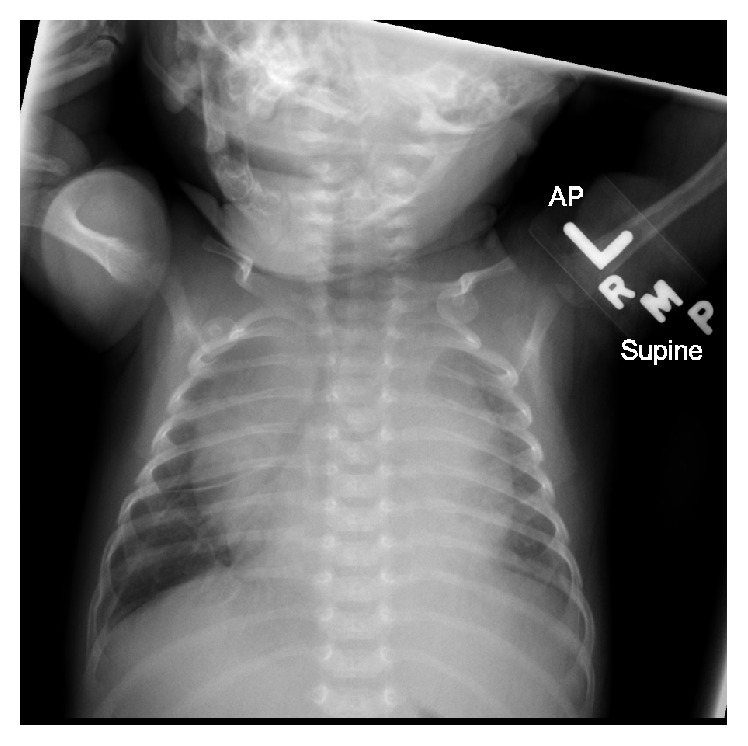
Anterior-posterior (AP) chest x-ray demonstrating marked enlargement of the cardiothymic silhouette.
